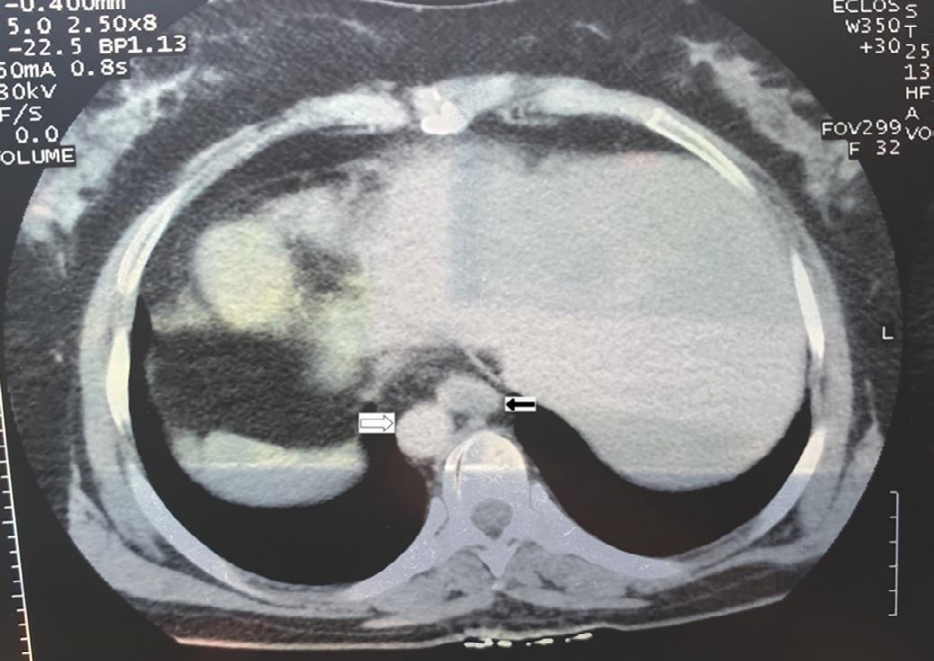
CT abdomen showing aorta on the right side (empty arrow) and inferior vena cava on left side (solid arrow).
